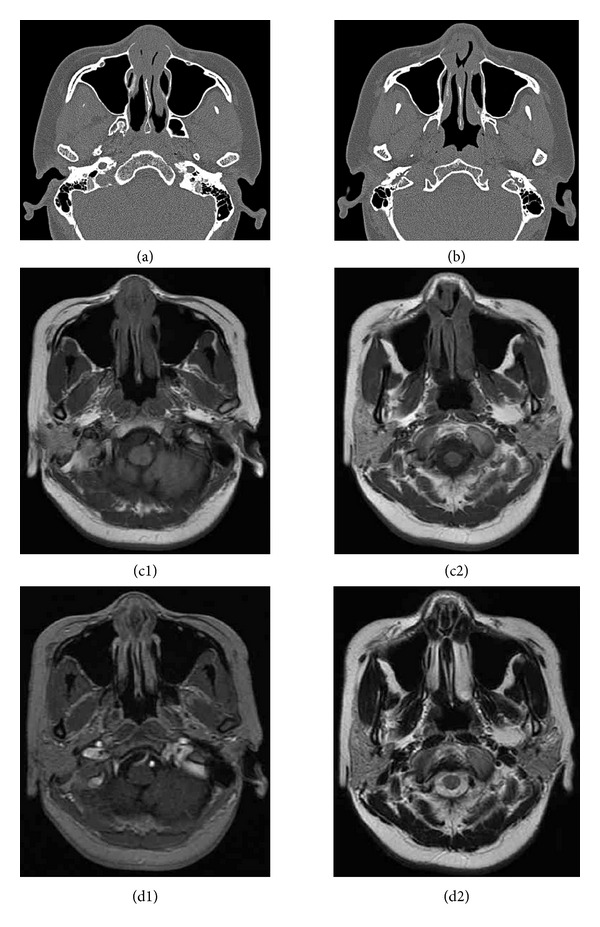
(a) The lesion appears to have homogeneous isodensity to gray matter on the nonenhanced CT. (b) The anterior nasal septum perforation was identified with its size approximately 1.3∗1 cm. (c1, c2) On the T1-weighted image, the anterior part of the septum and adjacent lateral nasal wall appear to be isointense to gray matter. (d1, d2) On the T2-weighted image, the anterior septum and bilateral headend of inferior turbinate are hypointense.
